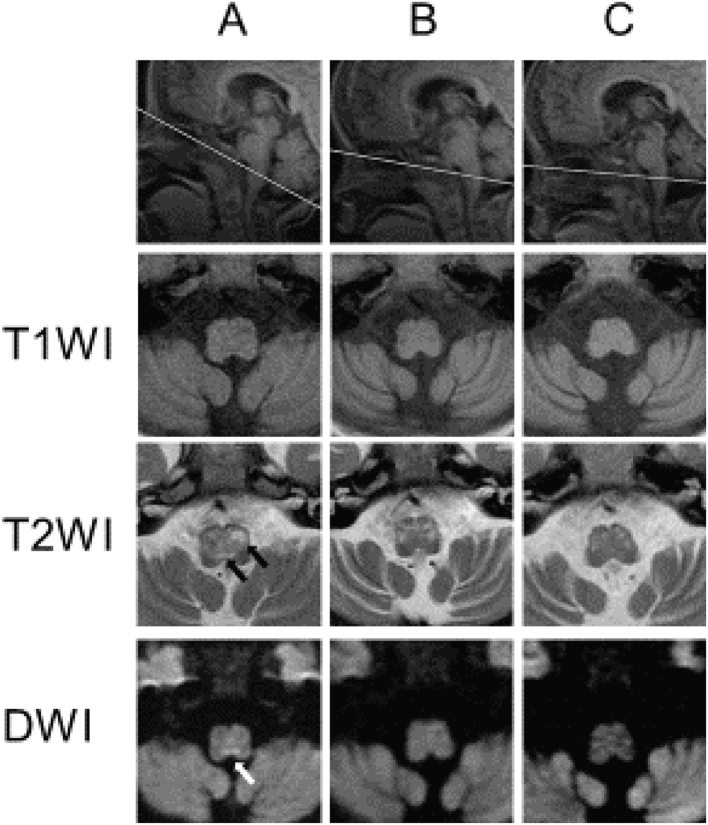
T2-weighted magnetic resonance images of the brain taken at the age of three years show increased signal intensity in the rostral and dorsal portion of medulla oblongata (black arrows) and diffusion weighted magnetic resonance images show high intensity signal in the dorsal part of medulla oblongata (white arrow) (A). T2-weighted MRI scans at the age of four years show slightly diminished high signal intensity of rostral portion of medulla oblongata and nearly complete disappearance of high intensity signal in the dorsal portion of the medulla oblongata (B). T2-weighted magnetic resonance images at the five years show more regression of the high intensity signals in the dorsal portion of medulla oblongata (C).
